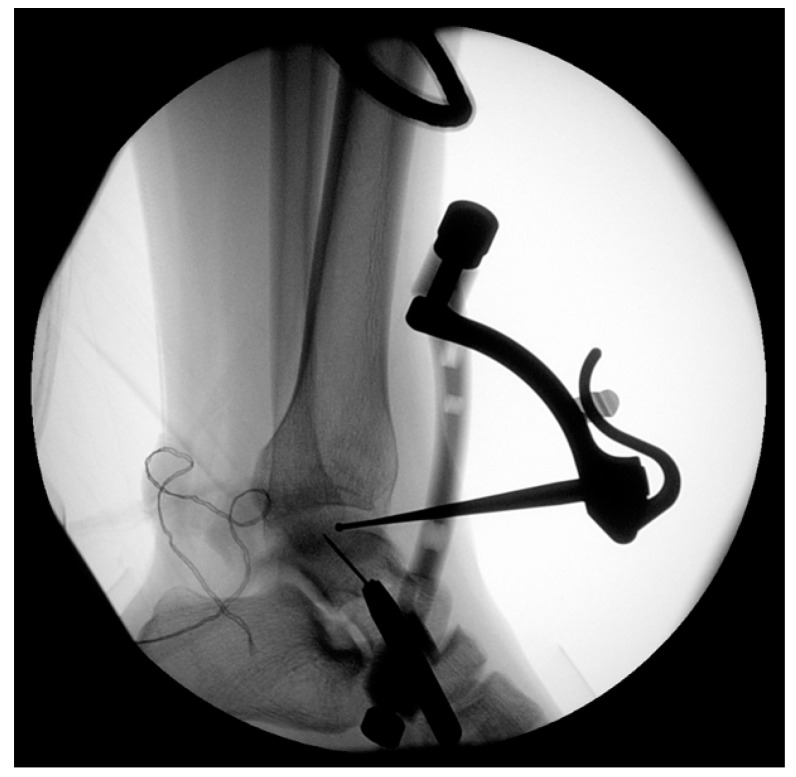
Intraoperative radiographic images. The direction and position of the guide pin is confirmed using a guide device. Lateral view of the right ankle.
